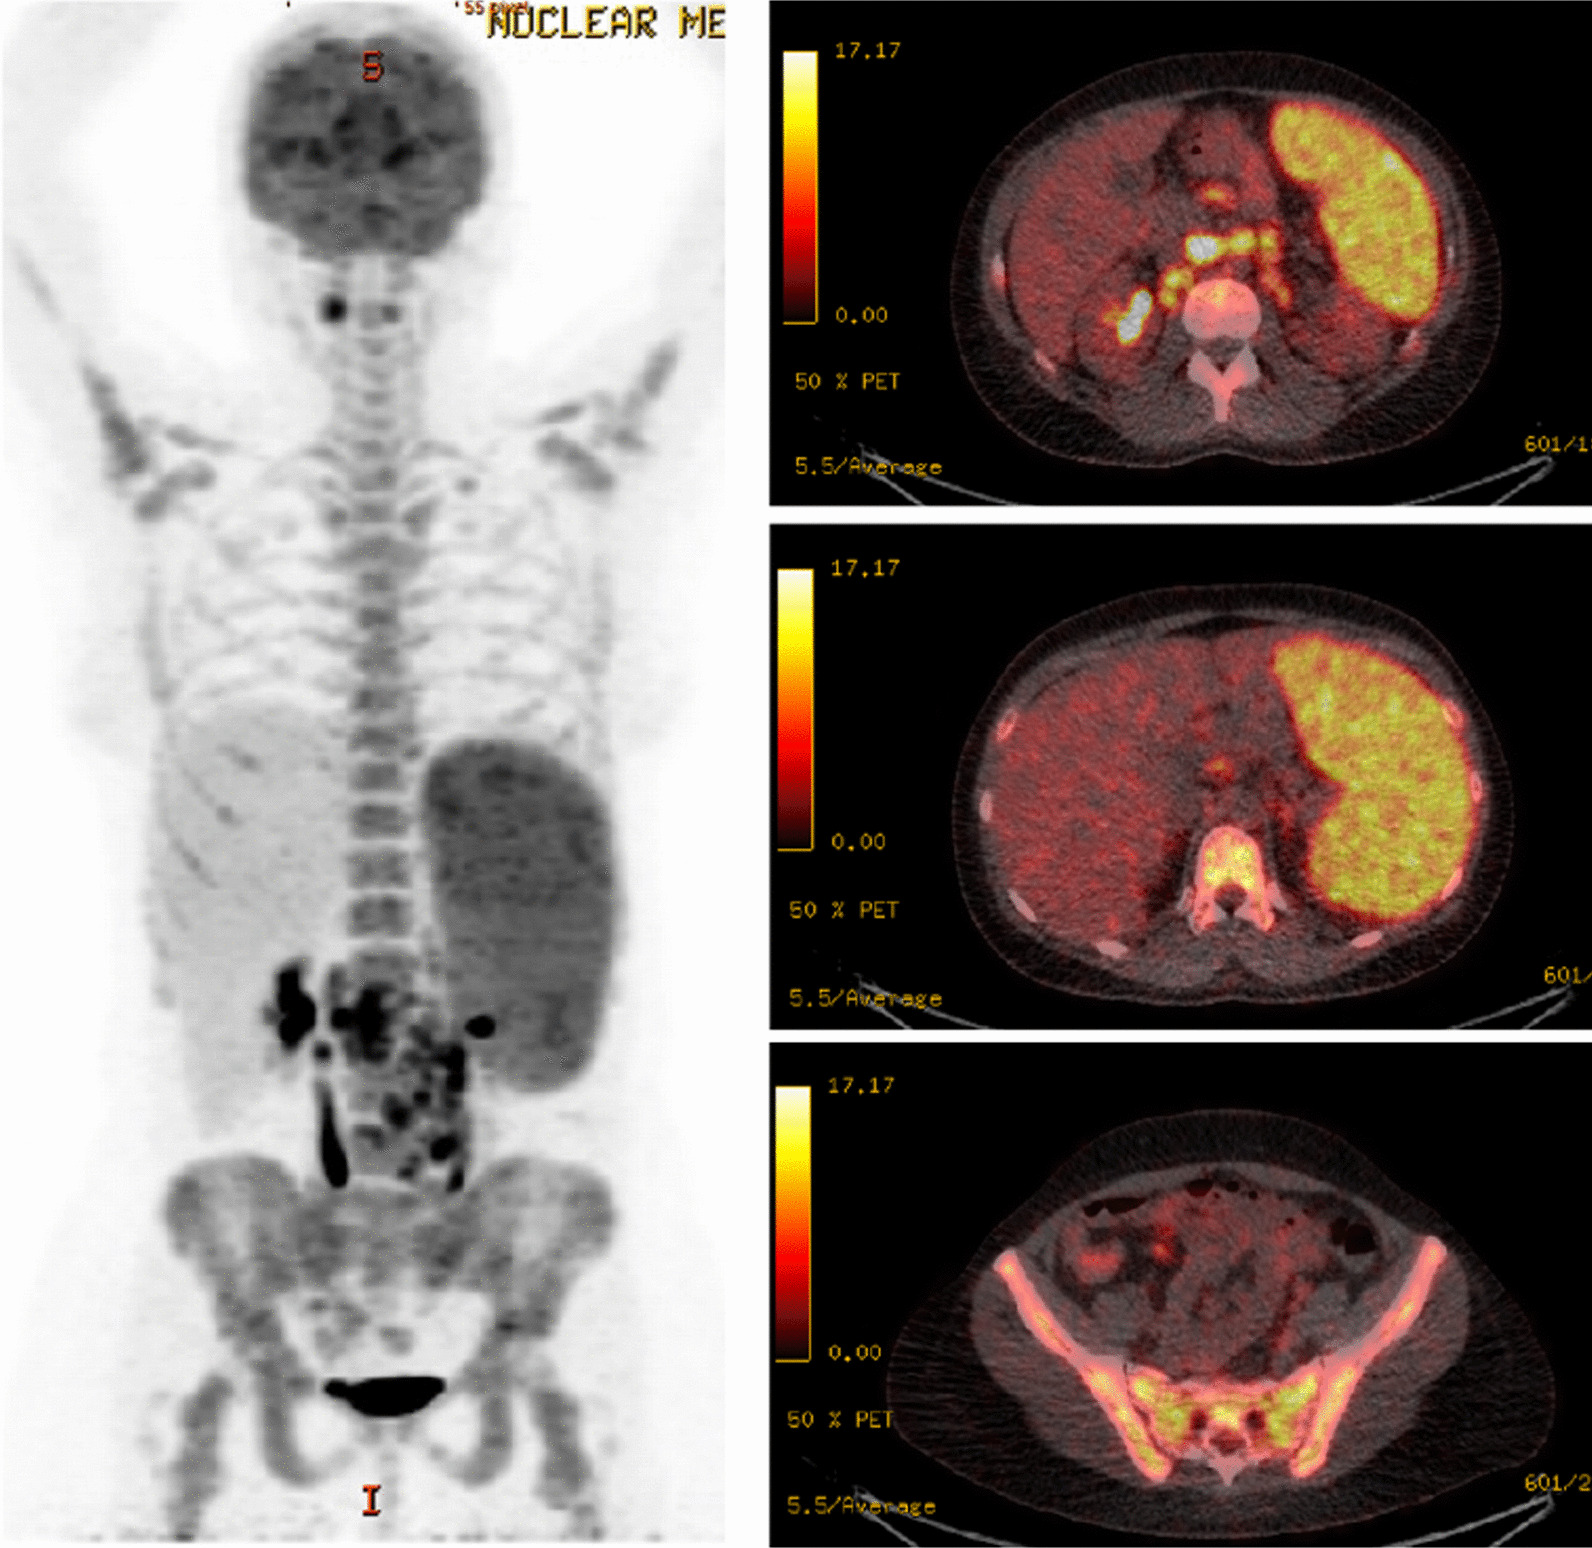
^18^FDG-PET analysis shows hypermetabolic lymphadenopathy and splenomegaly, together with enhanced tracer uptake in the small intestine, as well as a hypermetabolic bone marrow
